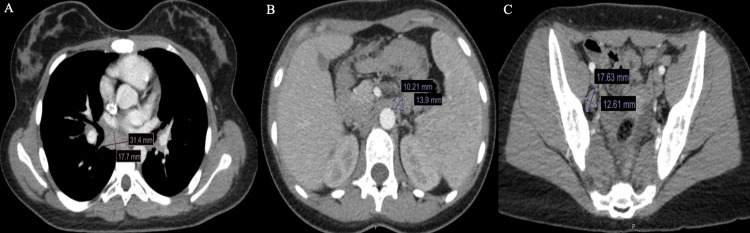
Computed Tomography (CT) of the Abdomen and Pelvis Multiple large mediastinal lymph nodes are seen. The largest lymph node measures 31.4 × 17.7 mm at the subcarinal level (A). Another lymph node measures 13.9 × 10.21 mm at the preaortic level (B), and a third measures 17.63 × 12.61 mm at the right external iliac level (C).
